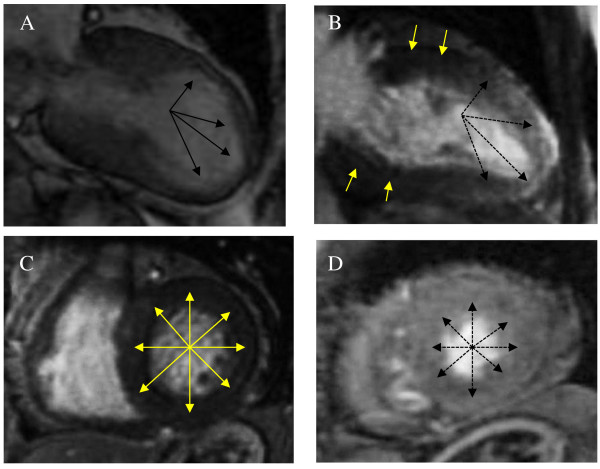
Cardiac magnetic resonance imaging in the sub-acute phase. (A) Cine CMR image during systole shows mid-apical dyskinesia as indicated by the arrows. (B, C, D) LGE inversion recovery images show that multiple diffuse patches were spread transmurally in the mid and apical portions, as indicated by the dotted arrows (B: vertical long axis, D: short axis at apical portion) compared with no LGE in the basal portion as indicated by the yellow arrows (C: short axis at basal portion). CMR indicates cardiac magnetic resonance and LGE, late gadolinium enhancement.
